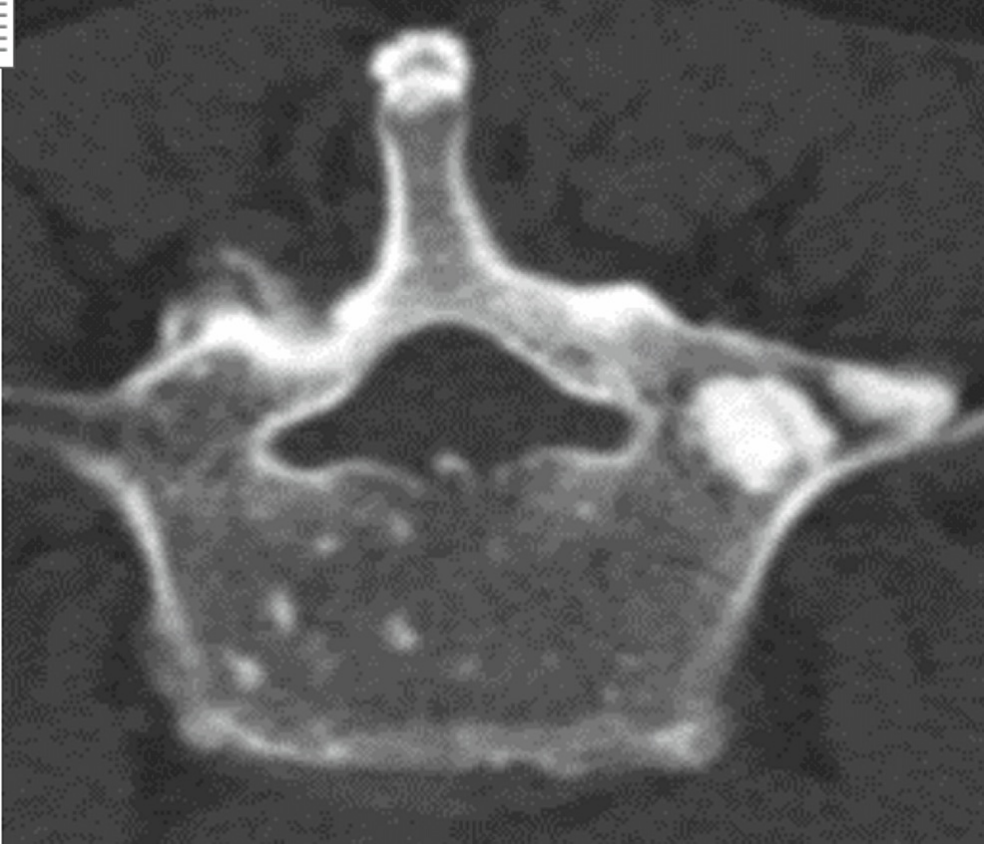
CT-guided bone marrow biopsy of the left iliac bone lesion CT: computed tomography
